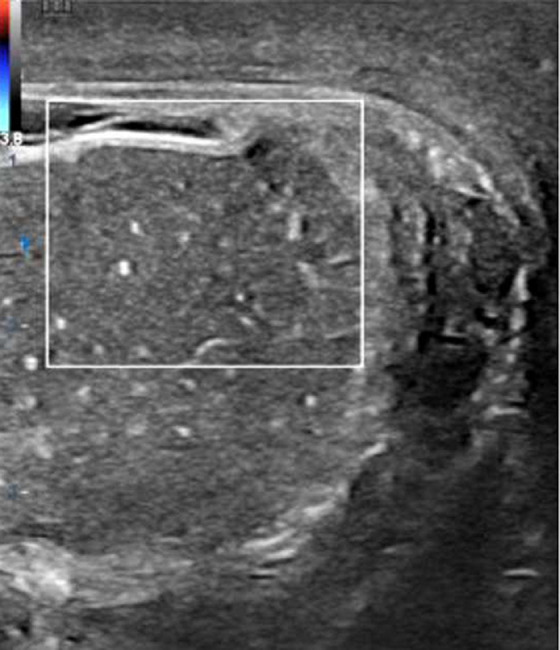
ultrasonography of the left testicle showing the absence of arterial vascularity
